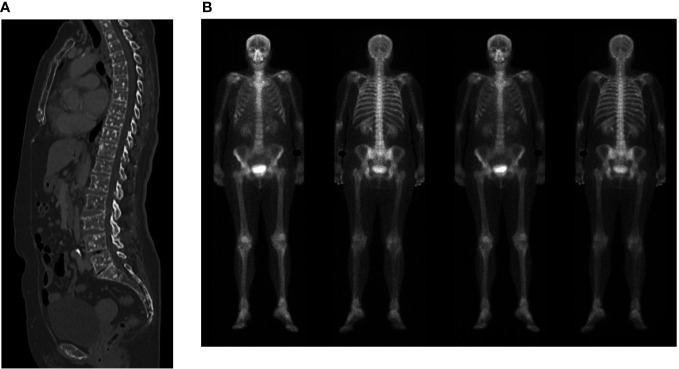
(A) Computed tomography scan showing multiple bone lesions. (B) Bone scan showing no evidence of bone metastases.
